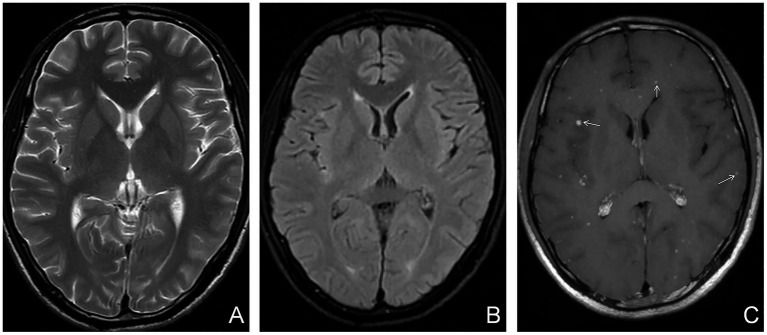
Non-edematous parenchymal tuberculoma. (A) Axial T2WI showing no abnormalities. (B) Axial T2-FLAIR image also showing no abnormal findings. (C) Axial contrast-enhanced T1WI image showing multiple homogeneously enhancing nodular lesions (white arrows) in the brain parenchyma, without hypointense perilesional edema.
